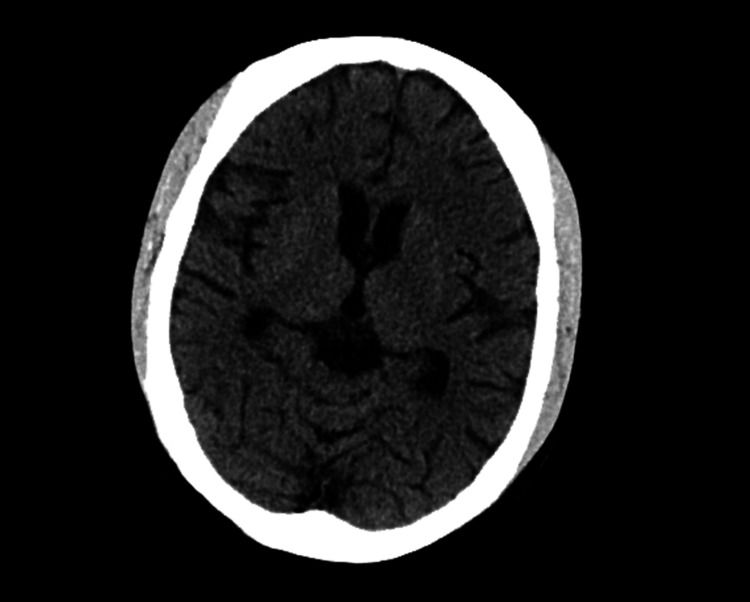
Axial view of CT head scan without contrast, reported to have no acute intracranial findings CT: computed tomography
